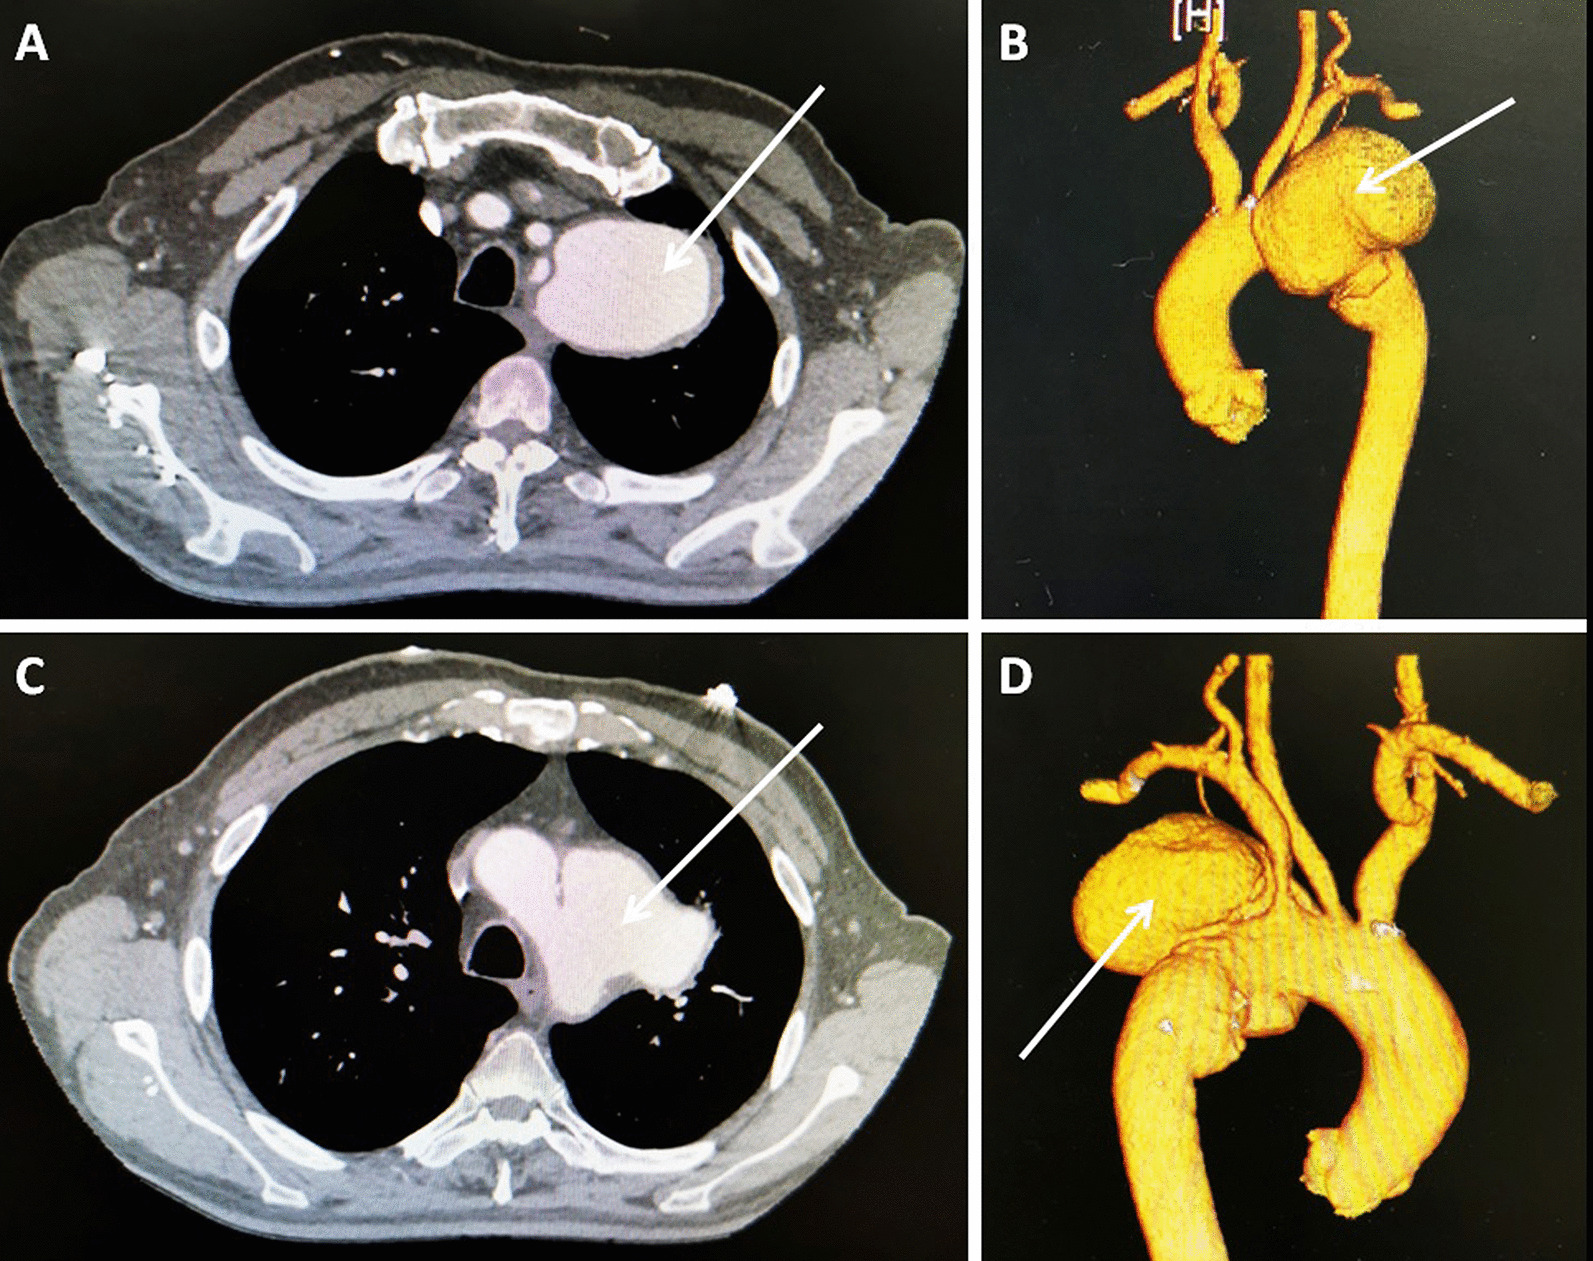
Preoperative computed tomographic scans showing a huge aortic pseudoaneurysm (58 × 56 mm, arrow indicating) involving the distal arch, proximal descending aorta, and left subclavian artery. A and C The computed tomography of aortic pseudoaneurysm. B Front view. D Posterior view
